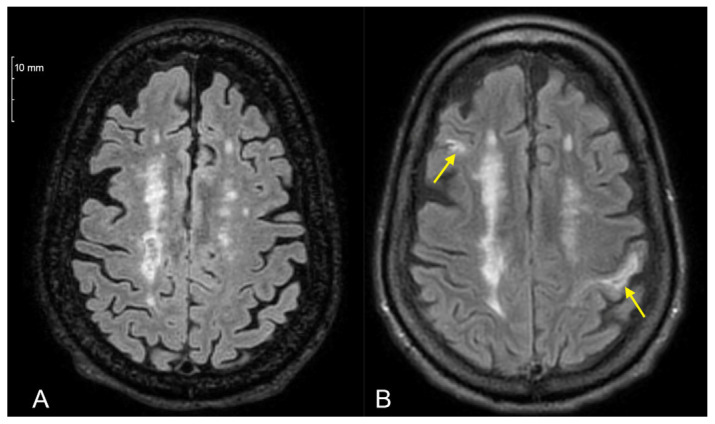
T2 fluid-attenuated inversion recovery magnetic resonance imaging of a patient (A) pre-index and (B) 18 months after pre-index date timepoint. The patient exhibited significant MS progression: apparent new lesions can be seen bilaterally in the left postcentral gyrus and right middle frontal gyrus (arrows).
